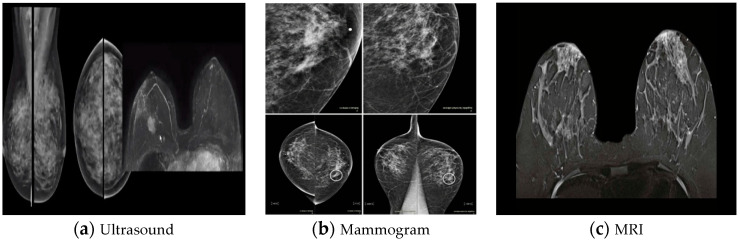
Medical imaging modalities for breast tissue: (a) ultrasound, (b) mammogram, and (c) MRI [10].
